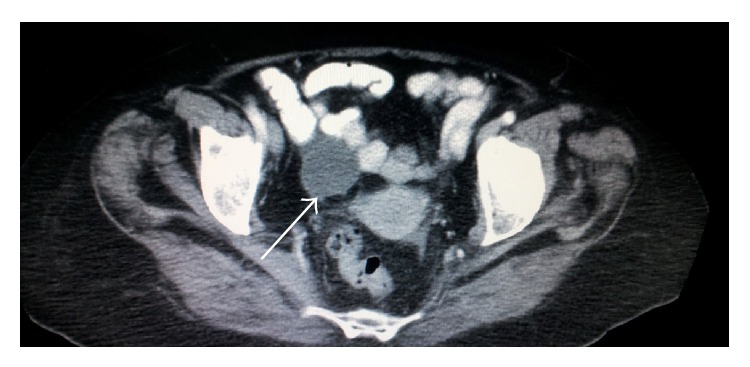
CT scan showing a swelling in the right iliac fossa (white arrow), misdiagnosed as ovarian cyst (second case).
